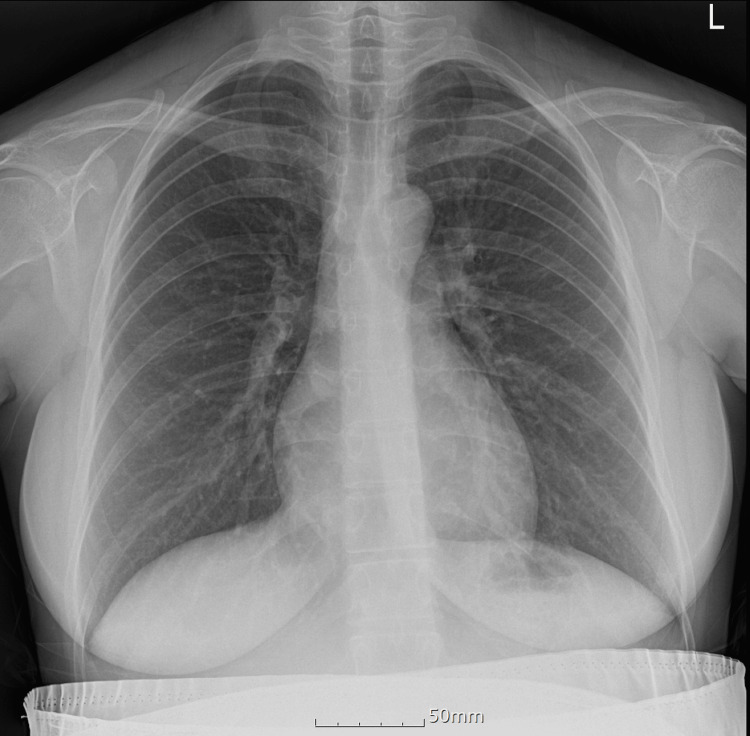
Preoperative chest radiography showing no abnormal findings
